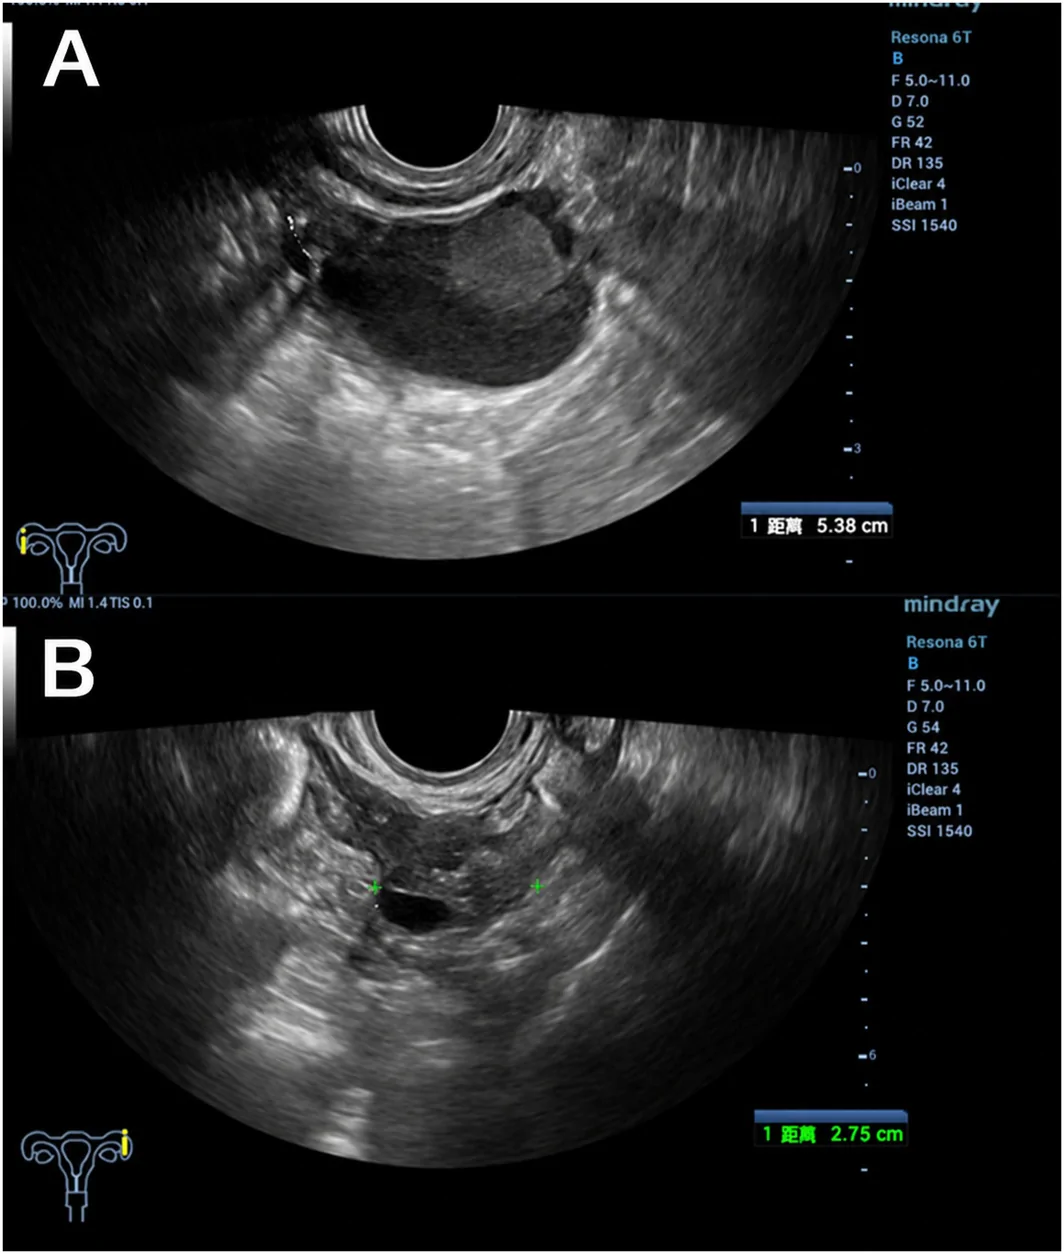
Ultrasound on February 14, 2025 (A, B). Post‐neoadjuvant ultrasound after sintilimab plus paclitaxel–carboplatin chemotherapy shows marked reduction of bilateral adnexal masses and disappearance of pelvic effusion.
